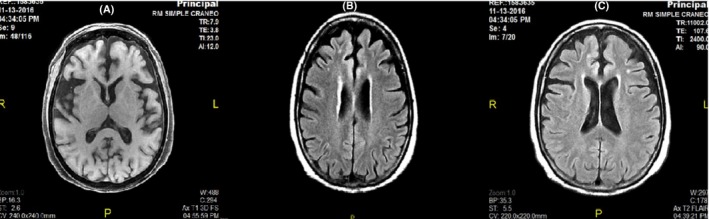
Axial‐brain MRI T1 FLAIR sequence that shows cortico‐subcortical atrophy of frontotemporal predominance and compensatory ventriculomegaly (A). T2 FLAIR sequence shows focal hyperintense subcortical lesions in frontal lobe and periventricular area, <1 cm in diameter, consistent with small‐vessel disease (B, C).
